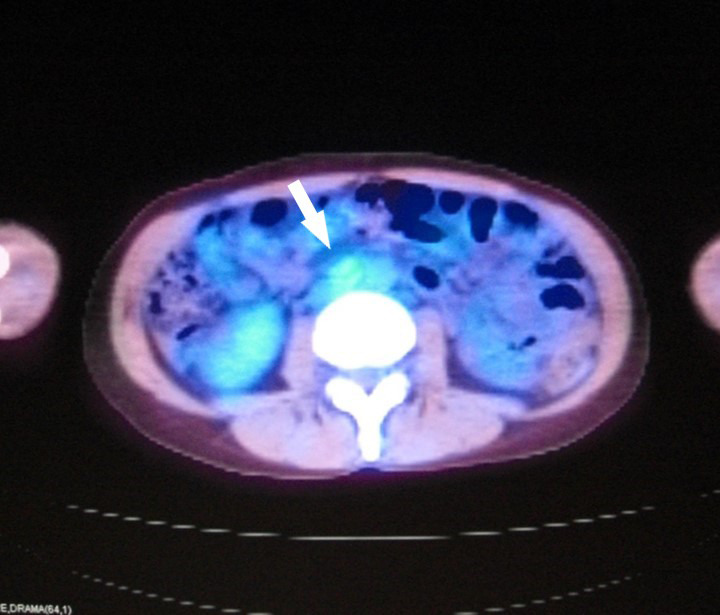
Swollen lymph nodes in the abdominal para‐aorta revealed on positron‐emission tomography and computed tomography (PET/CT: white arrow)
